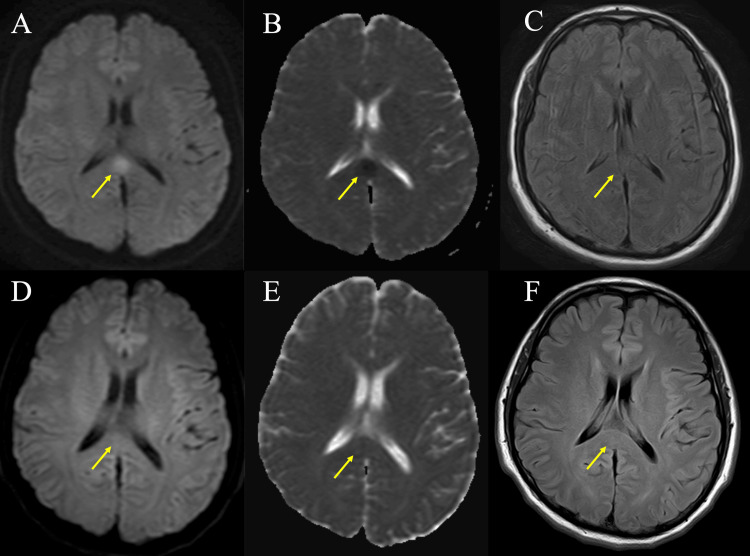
Case 1: MRI findings of CLOCCs (A) DWI shows a high-signal-intensity lesion in the splenium of the corpus callosum (yellow arrow). (B) The ADC map shows a corresponding low-signal-intensity lesion, indicating restricted diffusion (yellow arrow). (C) FLAIR imaging shows no significant signal abnormality in the corpus callosum (yellow arrow). (D-F) Follow-up MRI shows complete resolution of the corpus callosum lesion on DWI (D), ADC (E), and FLAIR (F). ADC, apparent diffusion coefficient; CLOCCs, cytotoxic lesions of the corpus callosum; DWI, diffusion-weighted imaging; FLAIR, fluid-attenuated inversion recovery
